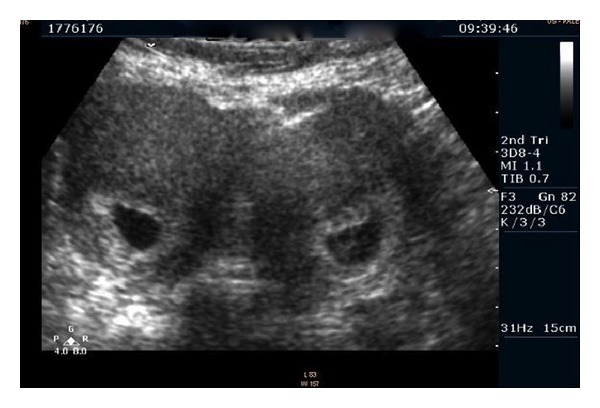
Two gestational sacs in coronal and sagittal view of 3D ultrasound.
